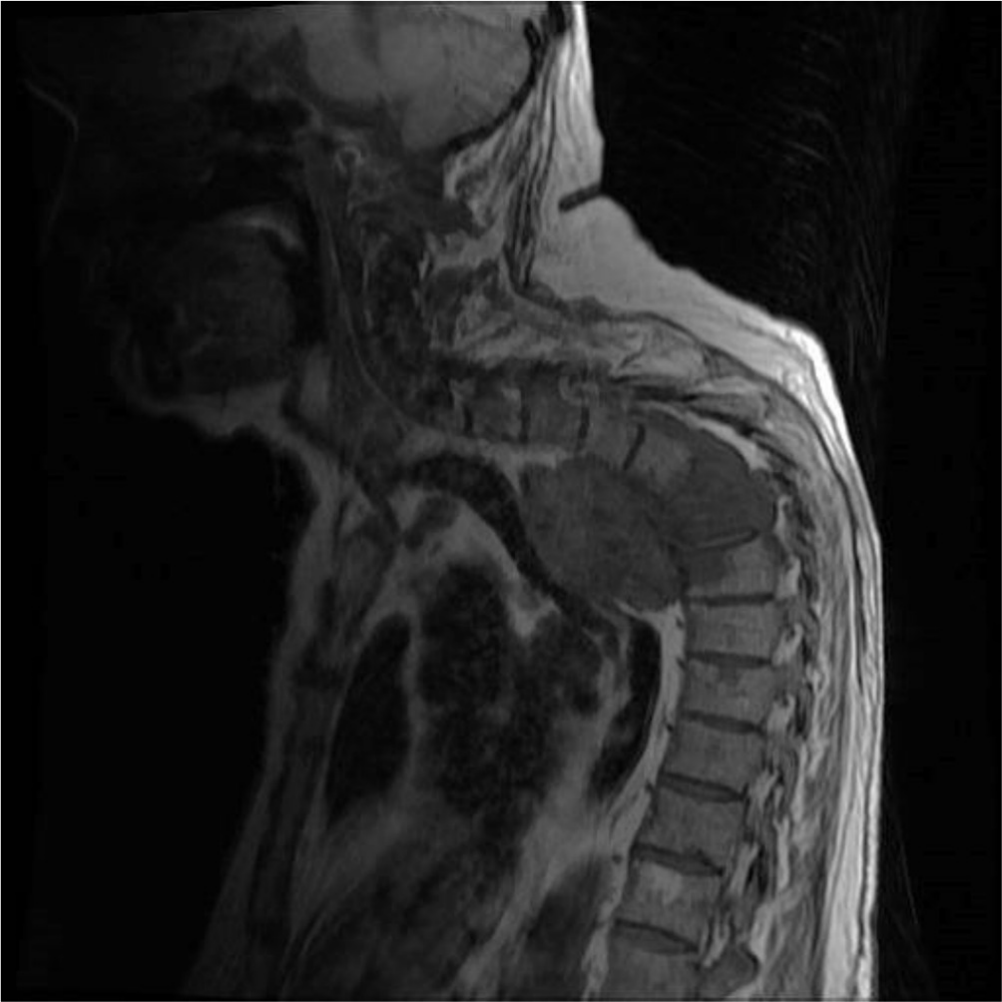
Axial slice of a CT-guided biopsy of a pleural lesion through the posterior thoracic wall.
